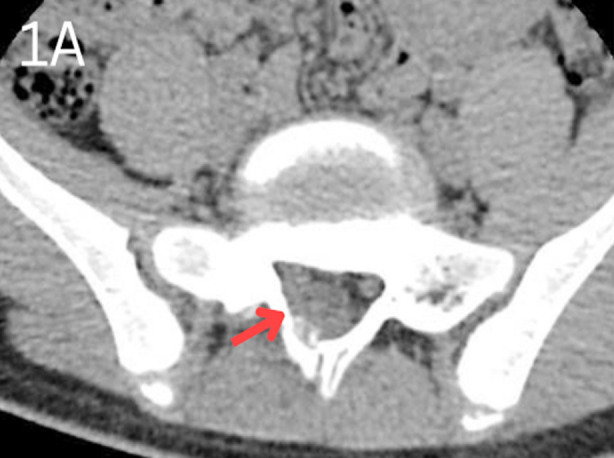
Contrast-enhanced computerized tomography (CE-CT) scan of sacrum and sacro-iliac joints. Red arrow indicates a well-defined lytic lesion of S1 lamina.
